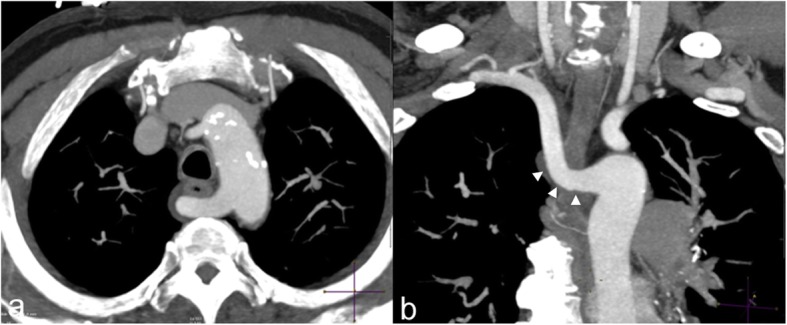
Aberrant right subclavian artery. Axial (a) and coronal (b) MIP thin reconstruction of a CT angiography of chest showing aberrant retroesophageal course of the right subclavian artery (arrowheads)
